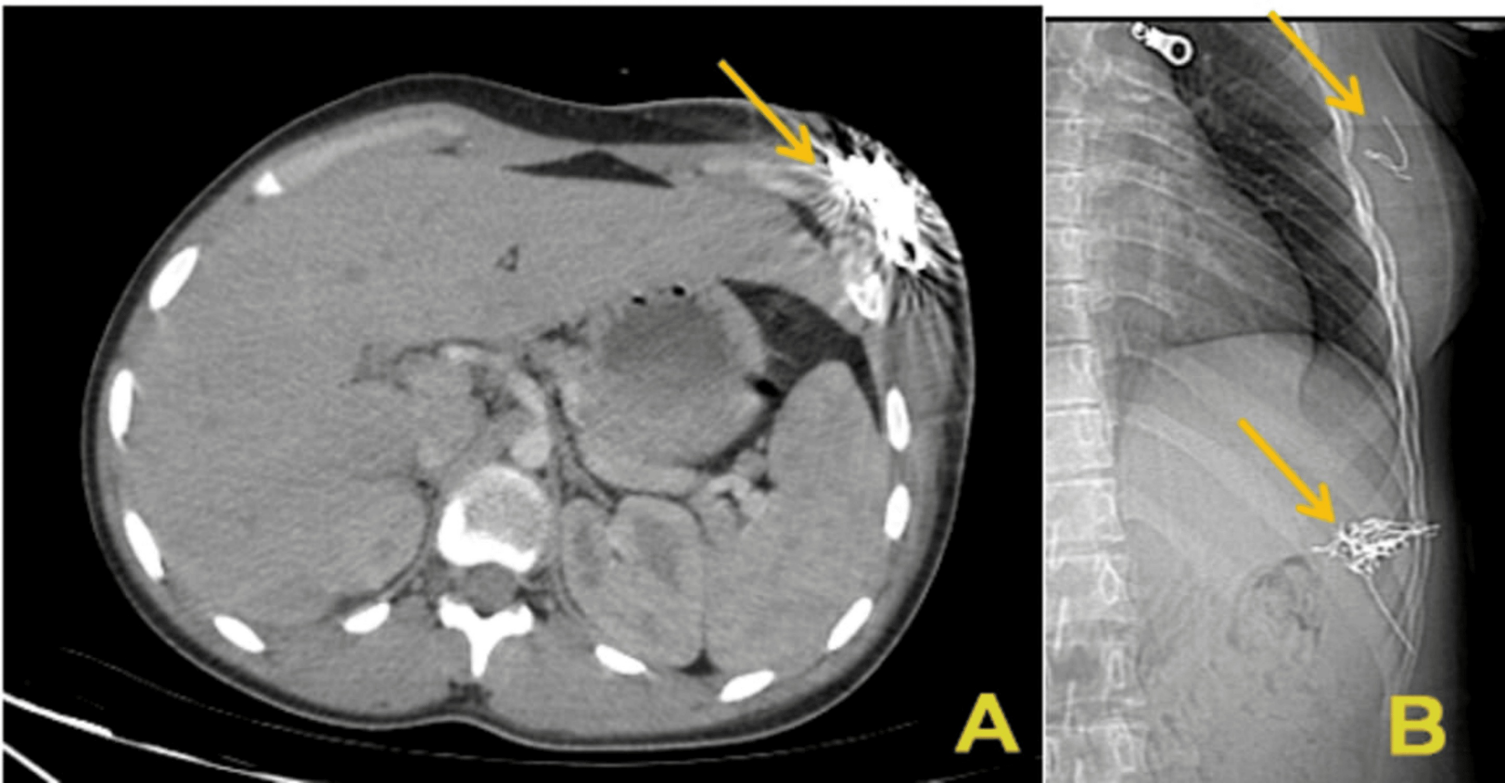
CT image (A) and radiographic image (B) showing the location of the foreign bodies, indicated by yellow arrows. A) Axial contrast-enhanced CT scan of the abdomen showing a cluster of hyperdense linear structures in the subcutaneous tissue of the left hypochondrium (yellow arrow), consistent with metallic foreign bodies. Beam-hardening artifacts are visible around the area, indicating the metallic nature of the objects. B) Lateral radiographic view of the thoracoabdominal region highlighting two separate collections of foreign bodies: one located in the left hypochondrium and another in the subcutaneous tissue of the left breast (yellow arrows). The radio-opaque nature and clustering suggest multiple metallic fragments, most likely inserted subcutaneously.
